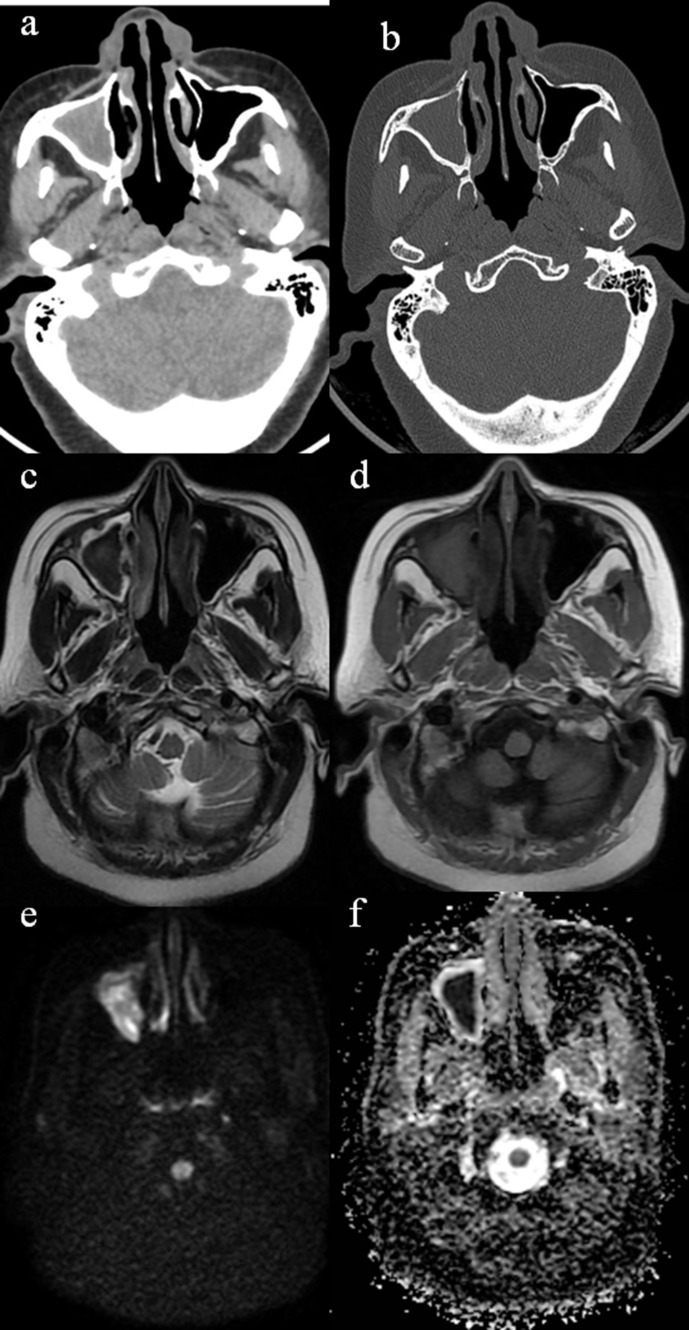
CT Axial soft tissue (a) and bone window images of mucormycosis shows complete opacification of right maxillary sinus (a) without any bone erosions (b). Axial MR images of same patient. Lesion shows hypointense signal on T2W images (c) and hyperintense signal on T1W images (d). Diffusion sequence images (e, b 1000; f, ADC map) show restricted diffusivity with low ADC value.
